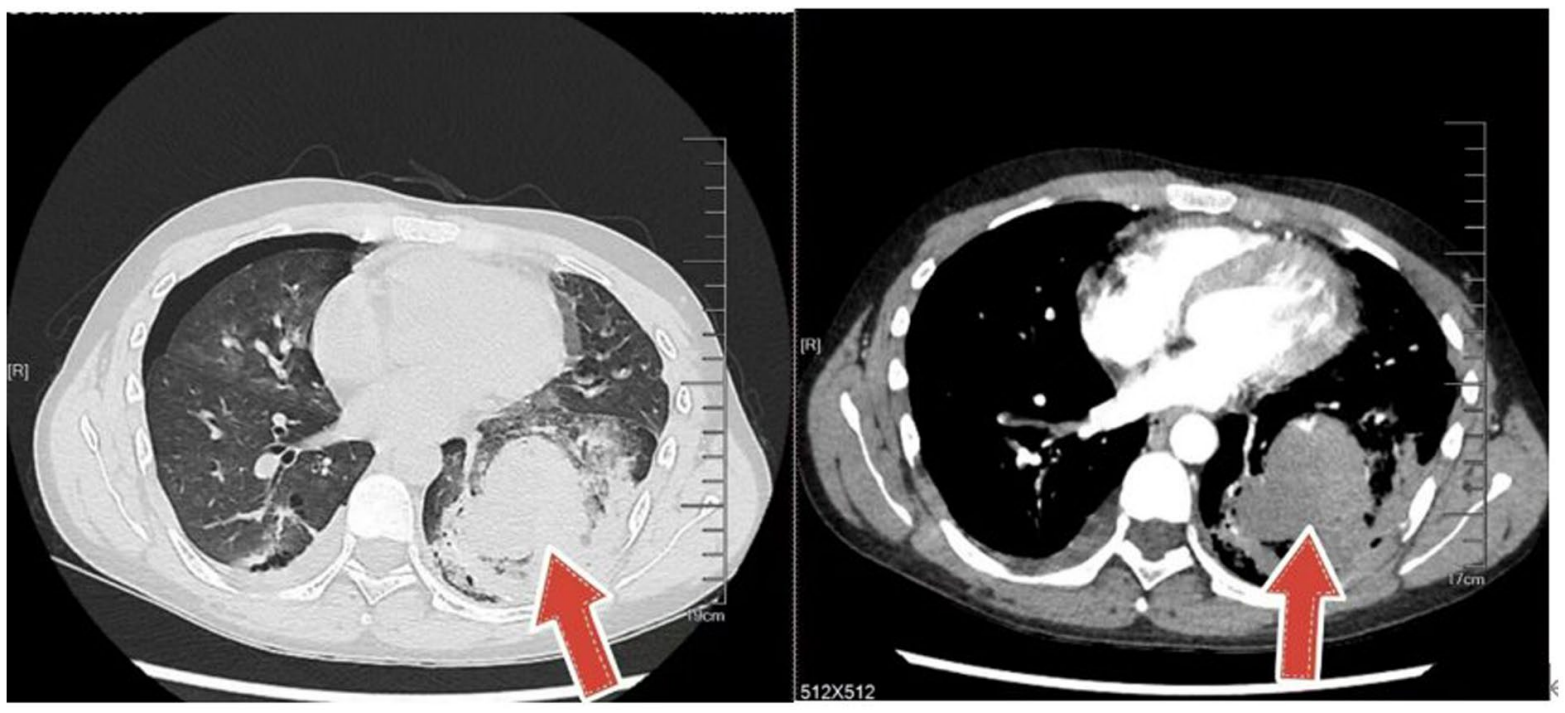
CT shows a massive hematoma in the left lung.
